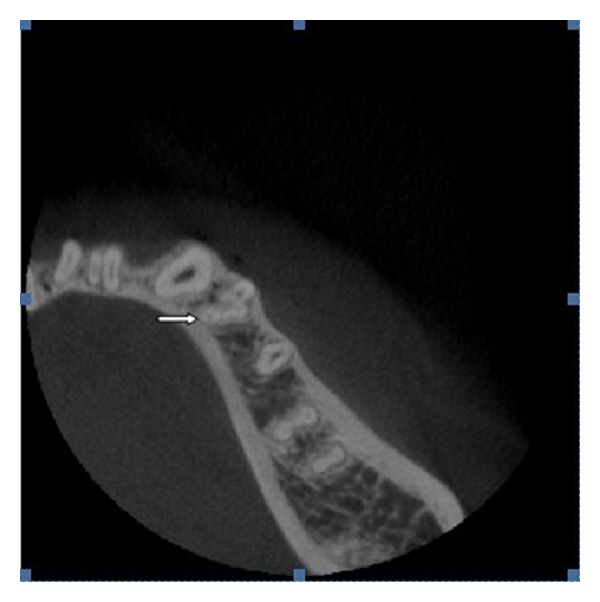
CBCT cross-sectional image of a mandibular first premolar (arrow), showing the spatial relation of a type VIII canal configuration and a deep mesial radicular groove (reprinted with permission from Yu et al. [11]).
